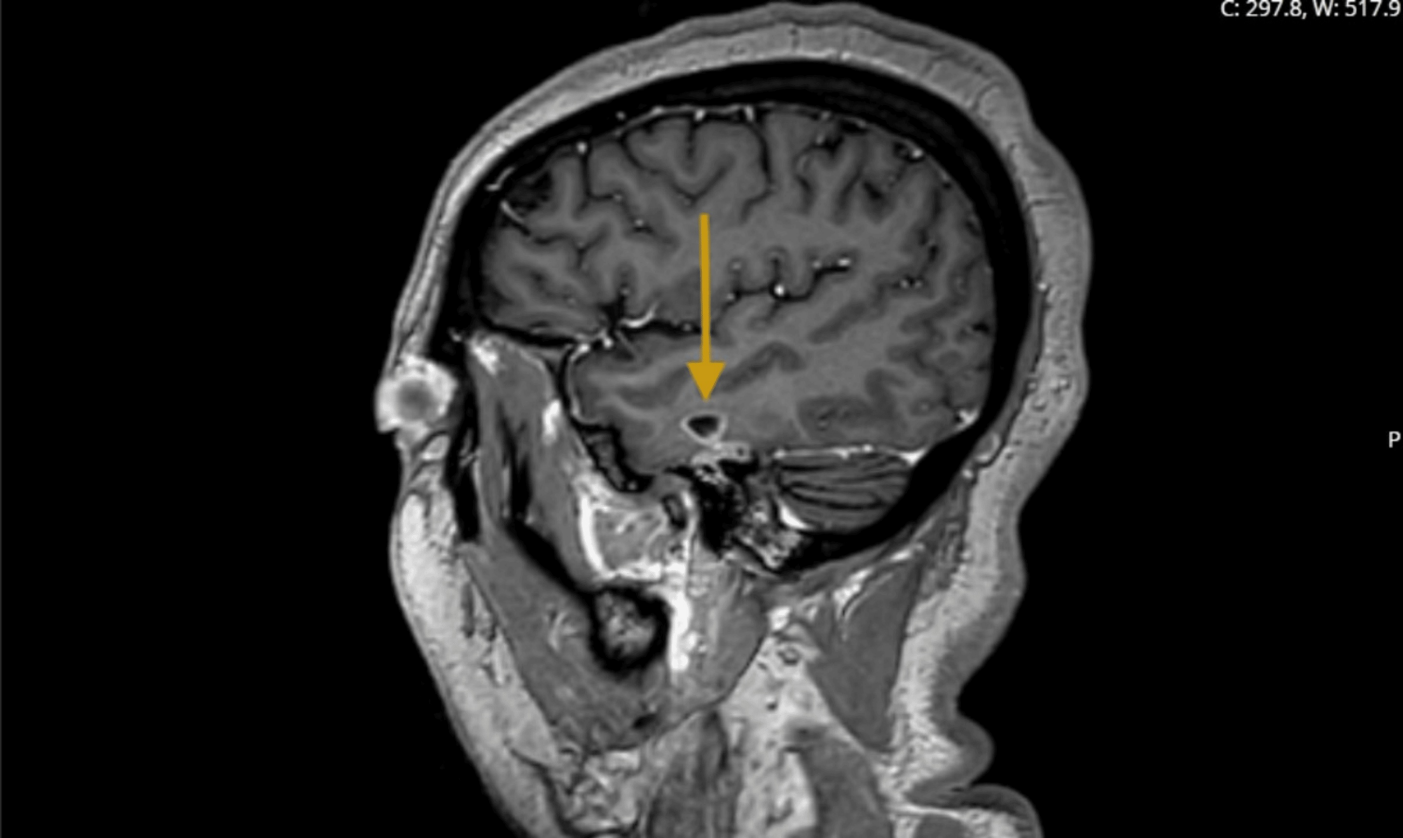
MRI of the brain with contrast (sagittal view) reveals a well-circumscribed, peripherally enhancing lesion within the left temporal lobe, corresponding to the measured 9 × 5 × 7 mm abscess.
